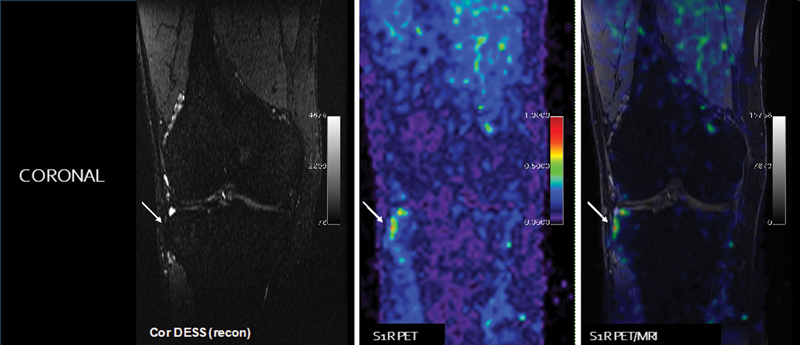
Young adult man with difficult to diagnose anterolateral knee pain > 1 year. Conventional knee magnetic resonance imaging (MRI) scans have been negative, and patient failed standard of care management until this point. Sigma-1 receptor positron emission tomography (PET)/MRI reveals increased radiotracer uptake at the insertion of the iliotibial band on Gerde's tubercle. This finally gave the referring physician good insight on how to move forward on what appears to be a traction enthesitis at this location. New therapeutic approaches, including local pain injections and physical therapy, led to eventual pain relief. (Courtesy of Sandip Biswal, MD, University of Wisconsin-Madison.)
